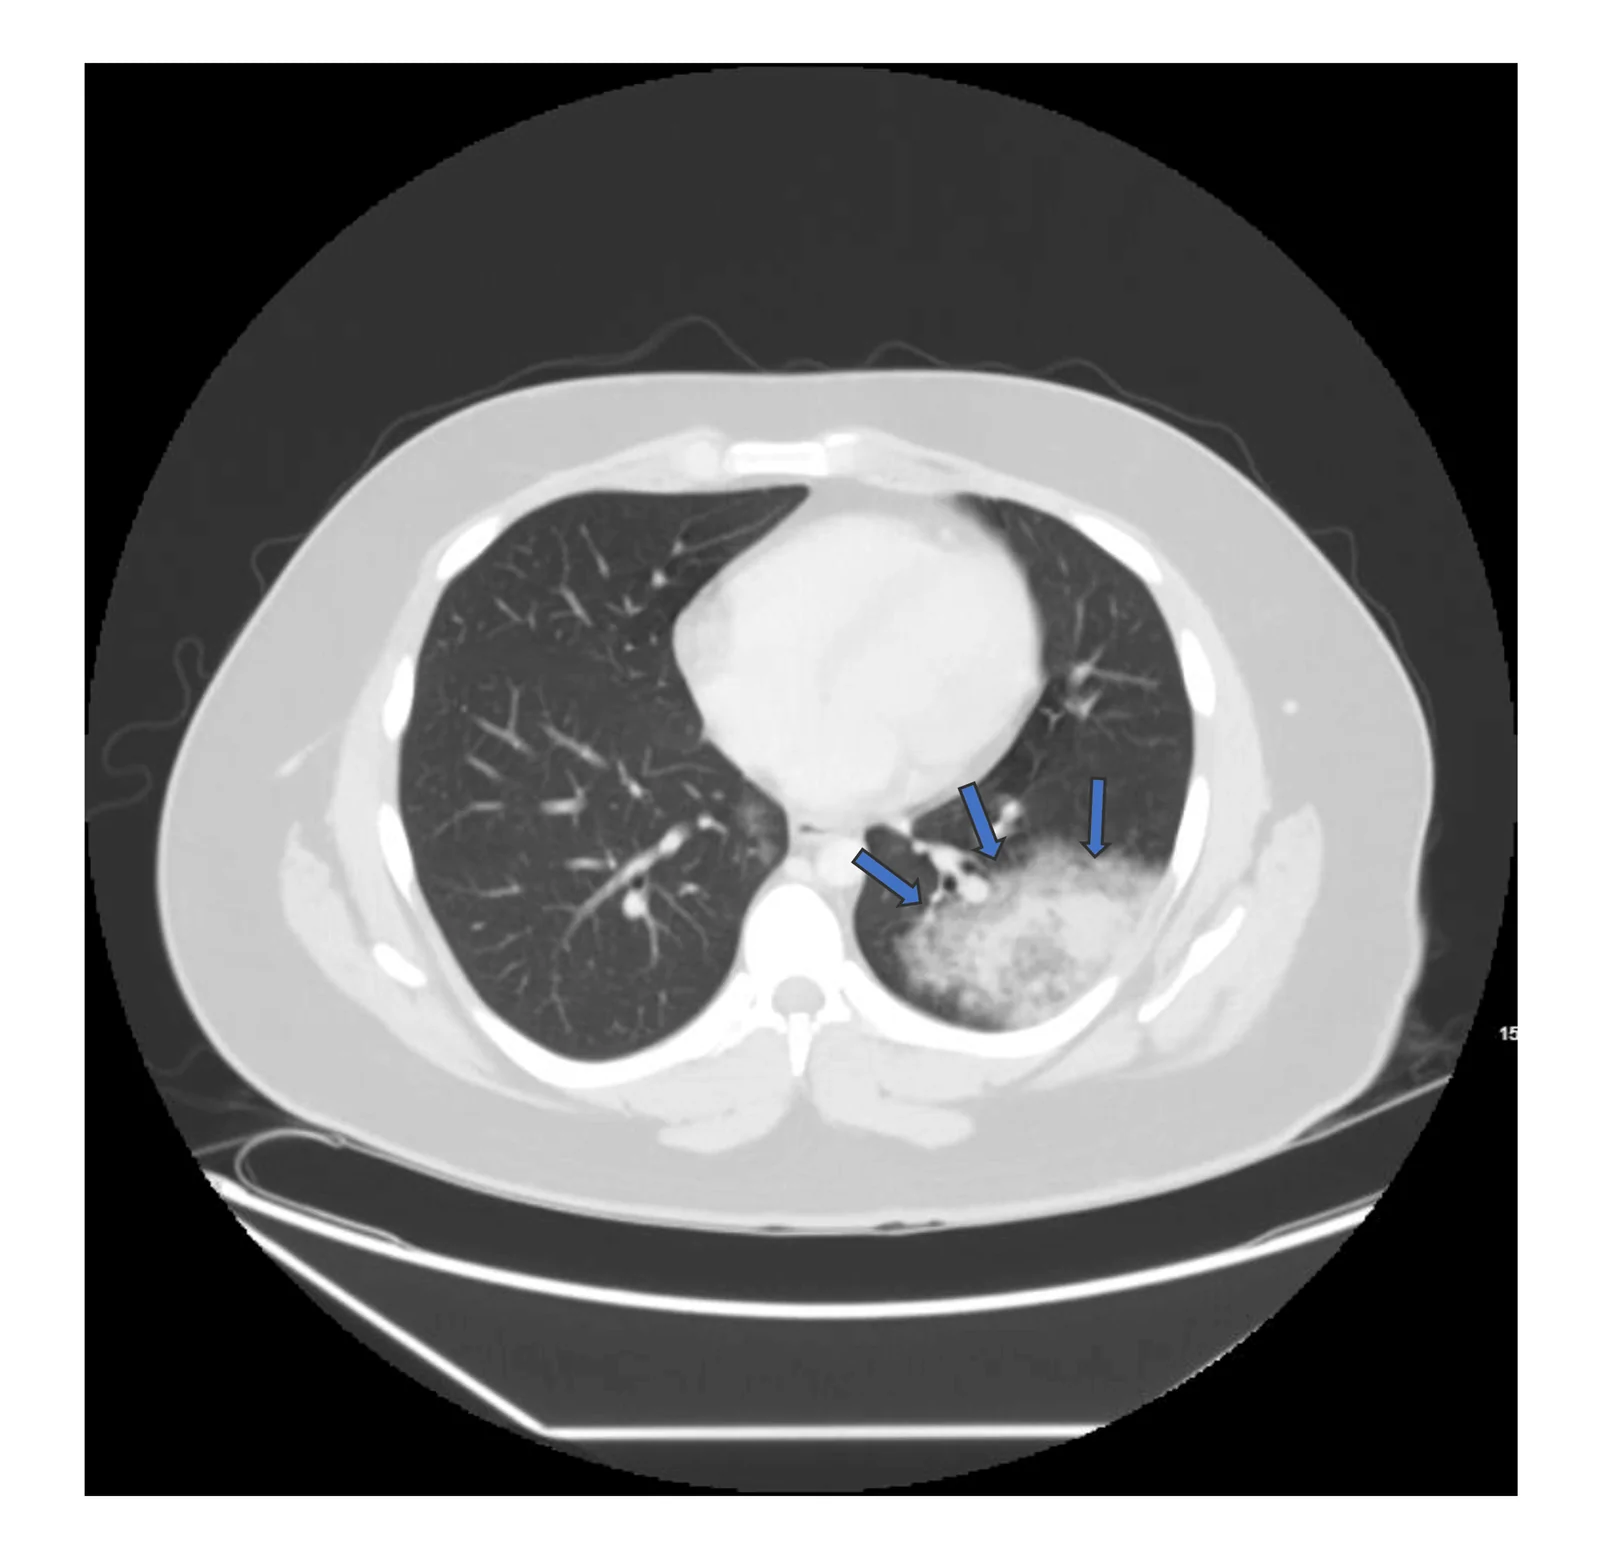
CT Scan of Chest, Abdomen and Pelvis with Contrast Demonstrating Left Lower Lobe Airspace Consolidation
